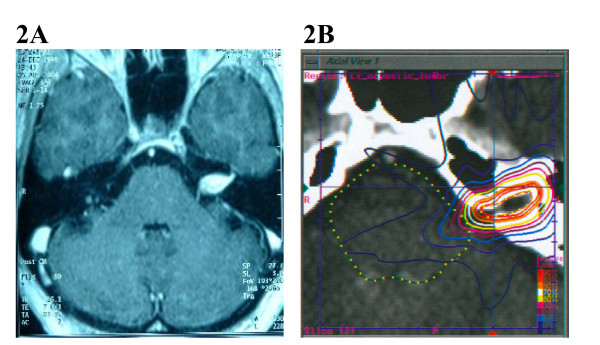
(A) A 77 year old woman presented ten years after craniotomy for acoustic neuroma resection with deafness. An axial MRI of the brain after gadolinium administration demonstrated radiographic progression of disease within the left internal acoustic meatus. (B) Planning CT scan with IV contrast. The patient was treated with 2500 cGy to the 84% isodose line in five stages.
